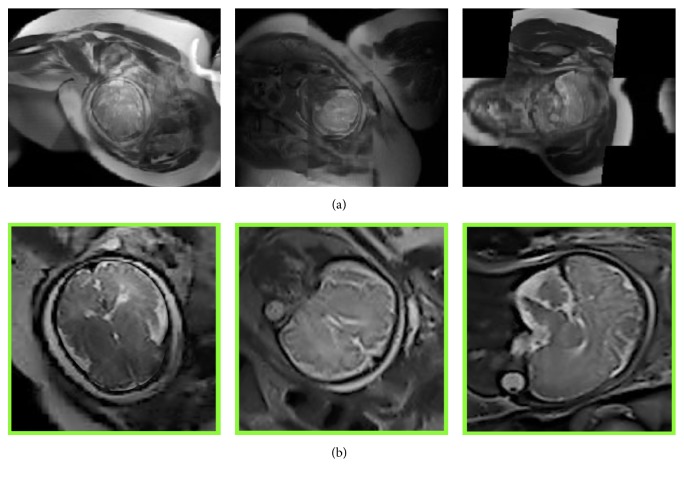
Three MRI scans reconstructed using raw MRI without brain localization and cropping (a). Inside the green colored frames (b), the same images were motion-corrected after automatic brain localization and cropping using the proposed method.
